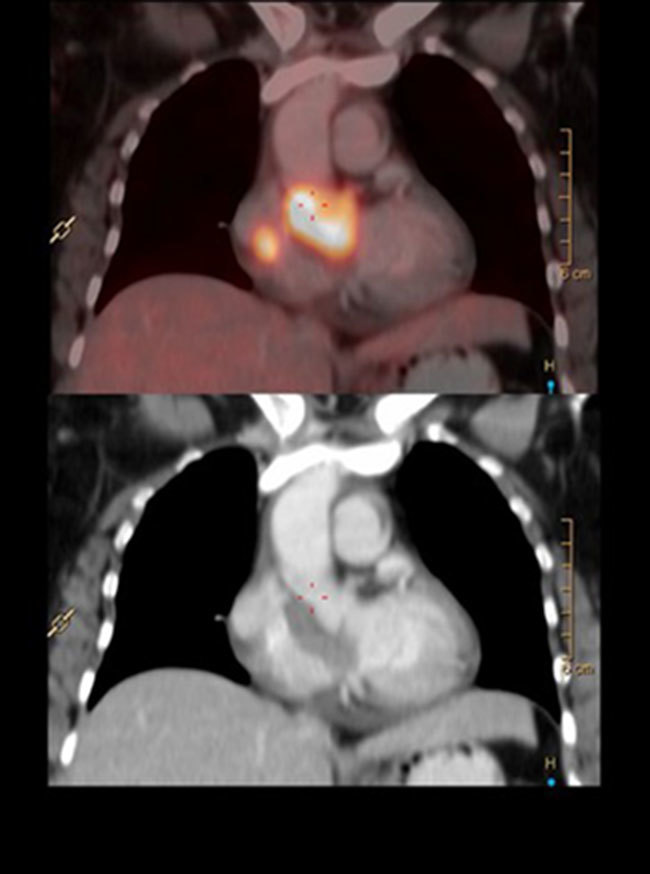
Sagittal sections of positron emission tomography (top) and CT image (bottom) showing cardiac metastasis.
